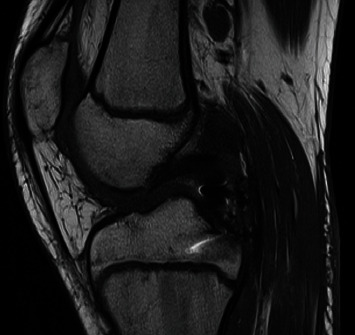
MRI of Case 1. The sagittal view 2 months after surgery shows the ligament inserted at its footprint but detached.
